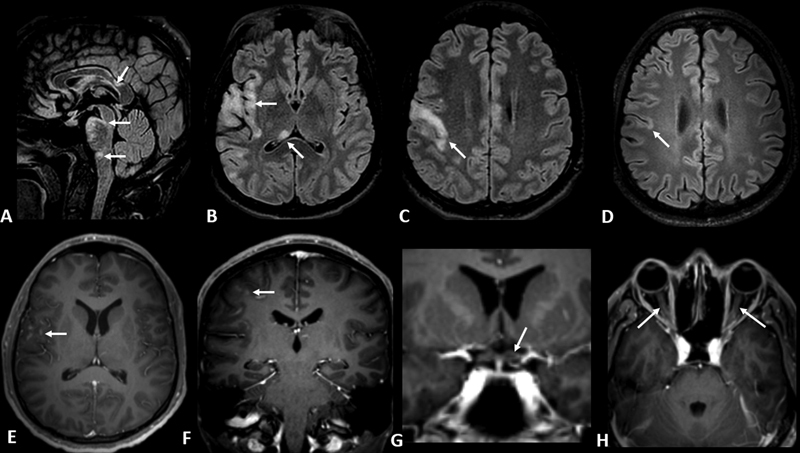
MRI findings of patient diagnosed with FLAMES (FLAIR-hyperintense lesions in anti-MOG-associated encephalitis with seizure). A 23-year-old previously healthy man developed severe headache, left sided hemiparesis, bilateral blurry vision, mental confusion and first epileptic seizure 3 weeks after receiving the Pfizer-BioNTech COVID-19 mRNA vaccine. Brain MRI images before steroid pulse therapy are shown: 3D-FLAIR with fat suppression (A-D); 3DT1 with fat suppression and after gadolinium administration (E-H). The arrows indicate lesions in the corpus callosum, brainstem, and cortex. Lesions are observed with perivascular CLIPPERS (chronic lymphocytic inflammation with pontine perivascular enhancement responsive to steroids) in image A, and cortical lesions with contrast enhancement in images E and F. There is peripheral enhancement of the chiasm (G) and bilateral optic nerves (H).
